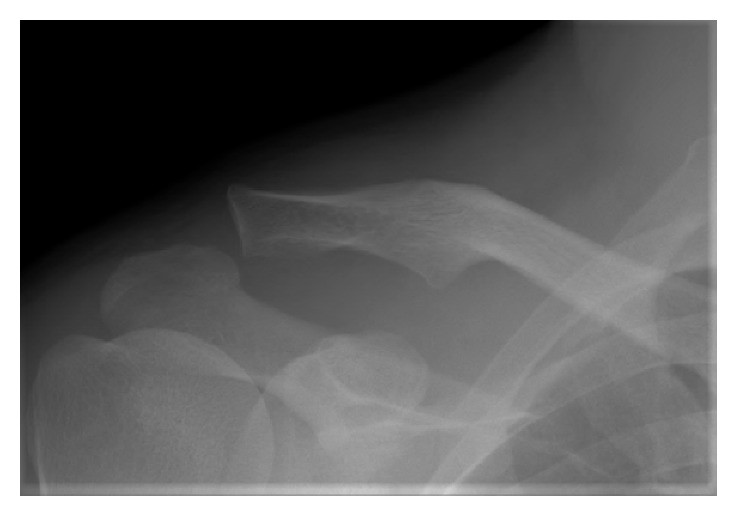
Case 1: images at the first presentation. A radiograph shows type III ACJ dislocation.
